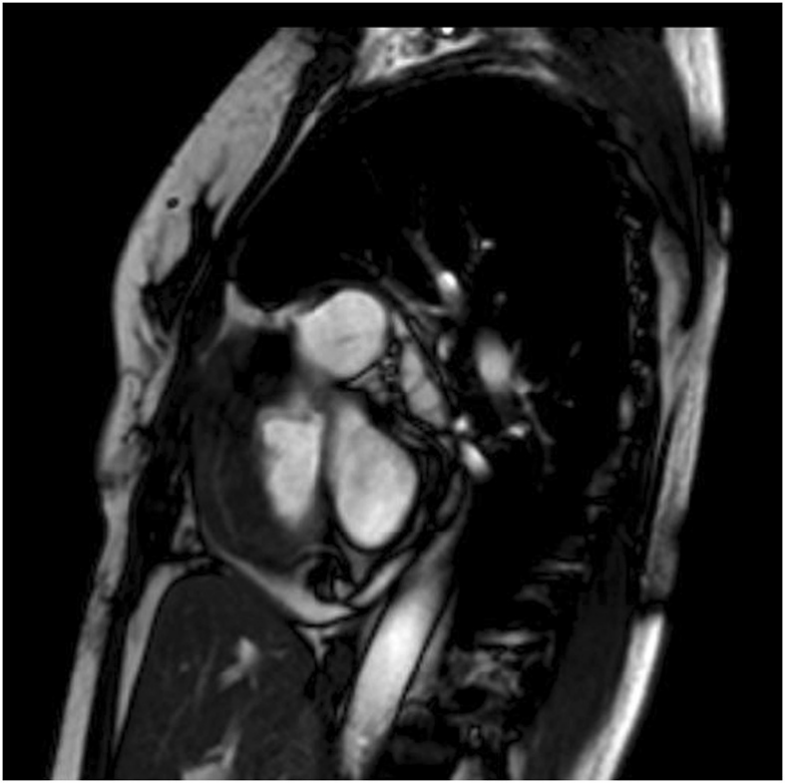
MRI T2 cuts, minor axis passing throughout the right atrium showing an irregular isosignal wall thickening of the side wall of the right atrium.
